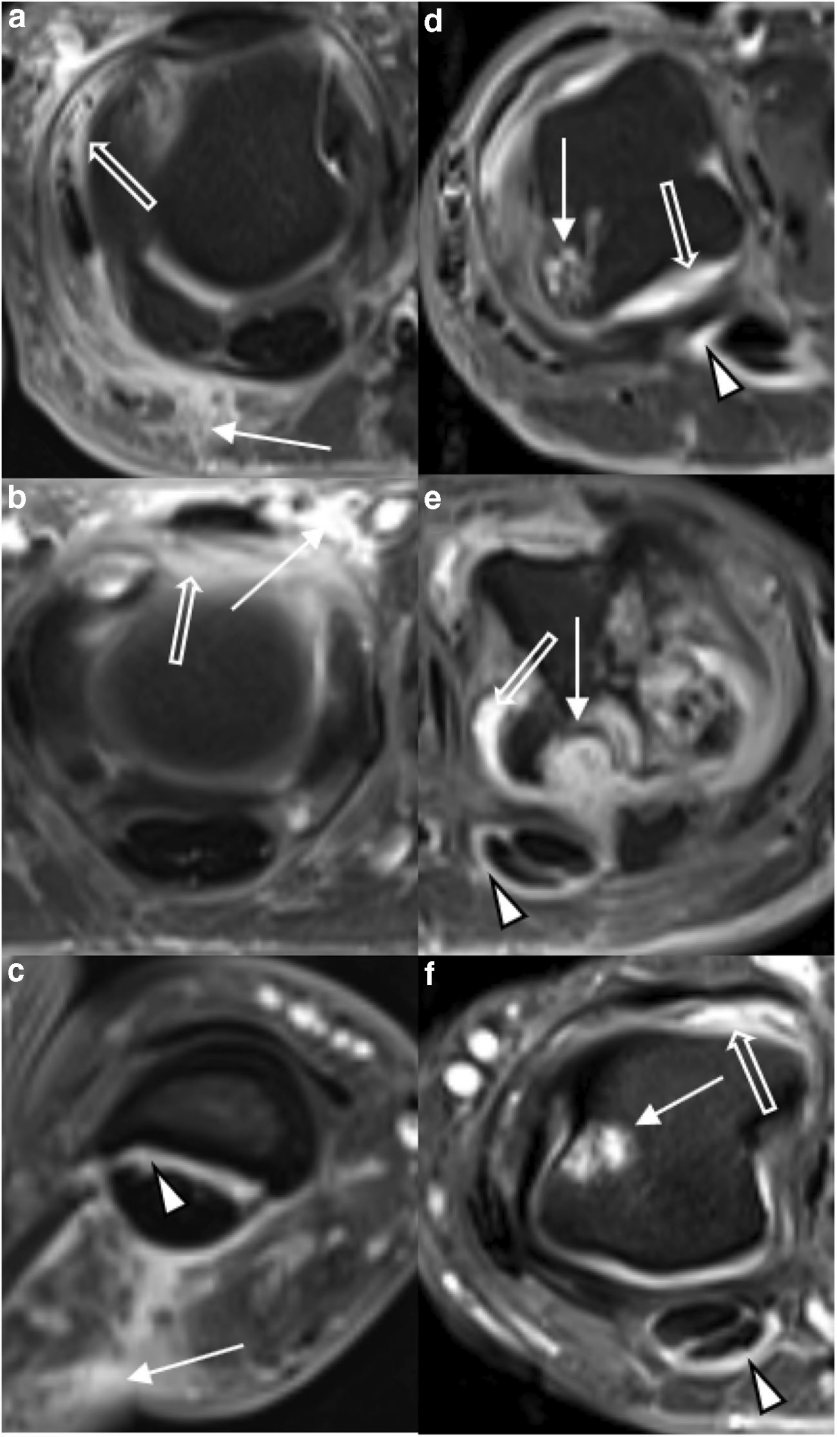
Detailed view of representative MRI findings in PsA and RA. Transversal T1w fat-saturated contrast-enhanced sequences of selected MCP joints of three patients each with PsA (a–c) and RA (d–f). a 34-year-old male. Severe periarticular inflammation (white arrow) with additional mild synovitis (open arrow). b 42-year-old female. Severe dorsal periarticular inflammation (white arrow) with synovitis (open arrow). c 44-year-old female. Severe periarticular inflammation (white arrow) with corresponding mild flexor tenosynovitis (arrowhead). d 39-year-old male. Widespread synovitis (white arrow) with moderate flexor tenosynovitis (arrowhead) and large bone erosion (white arrow). e 41-year-old male. Multiple large bone erosions (white arrow) and severe synovitis (open arrow) with mild flexor tenosynovitis (arrowhead). f 56-year-old female. Bone erosion (white arrow) with moderate flexor tenosynovitis (arrowhead) and moderate synovitis (open arrow). Note the absence of periarticular inflammation in d–f despite significant inflammatory joint changes at the joint level
